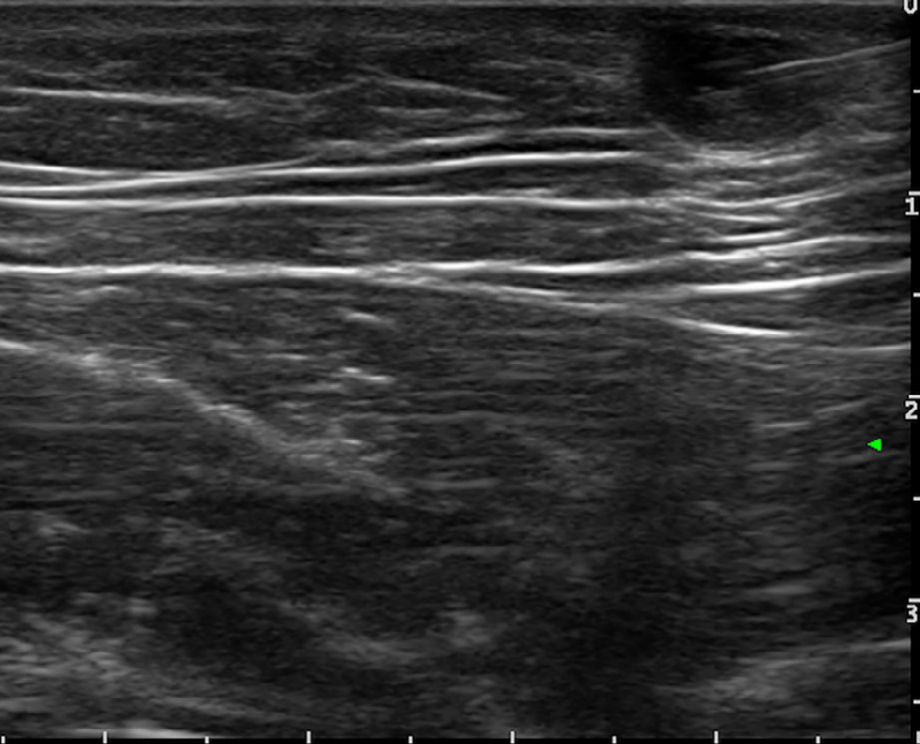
Ultrasound‐guided fine‐needle aspiration cytology of an in‐transit melanoma metastasis.
